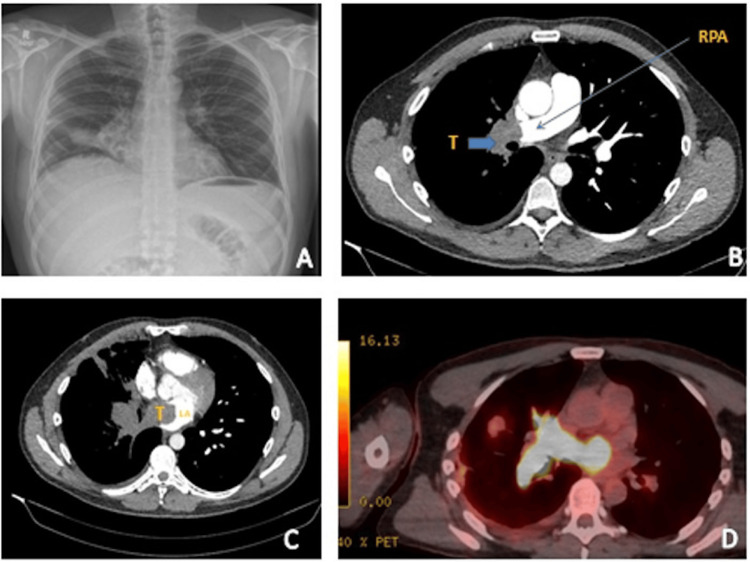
(A) PA chest X-ray showing abnormal opacification in the right hilar region. (B) CT Pulmonary Angiogram (CTPA) (transverse plane) showing complete occlusion of the right pulmonary artery (RPA) (arrow) with no blood flow to the middle and lower lobes.(C) A large thrombus (T) extending into the left atrium, occupying almost half of the left atrium, indistinguishable from a thromboembolism. (D) The thrombus is intensely 18F-fluorodeoxyglucose (FDG)-avid on PET-CT, confirming tumour invasion into the left atrium, with an SUVmax up to 41.
